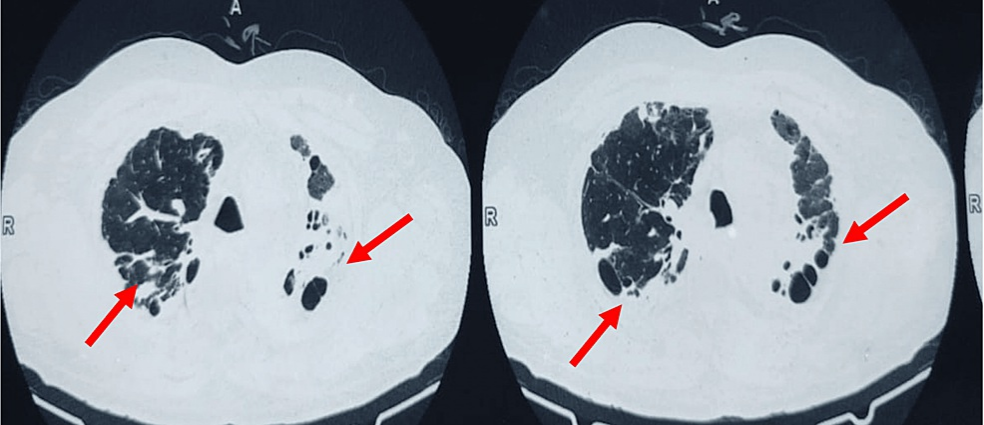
CT thorax shows bilateral bronchiectasis and fibro-cavitary (red arrow) changes in the upper zone with volume loss (post-tuberculosis)
